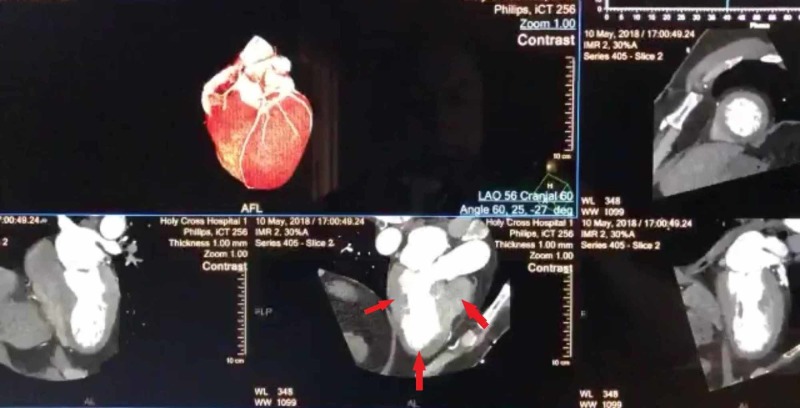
Coronary Computed Tomography Angiography with decreased perfusion in left ventricular apical segment and dyskinesia The red arrows show a still image of a coronary computed tomography angiography demonstrating decreased perfusion to the left ventricular apical segment with an estimated ejection fraction of 28%.
